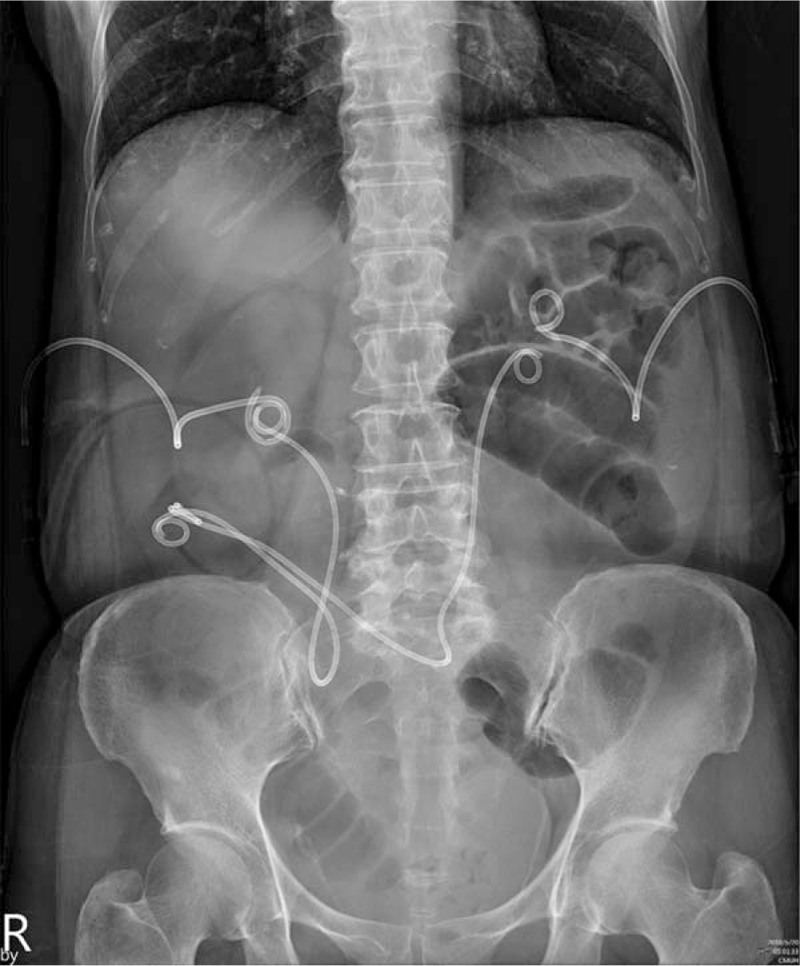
Abdominal X-ray of the patients showed a distended small bowel. Because of the high position of the stoma site, the bilateral ureters formed a U-shaped bend and lead to strangulation of the hernia loop.
